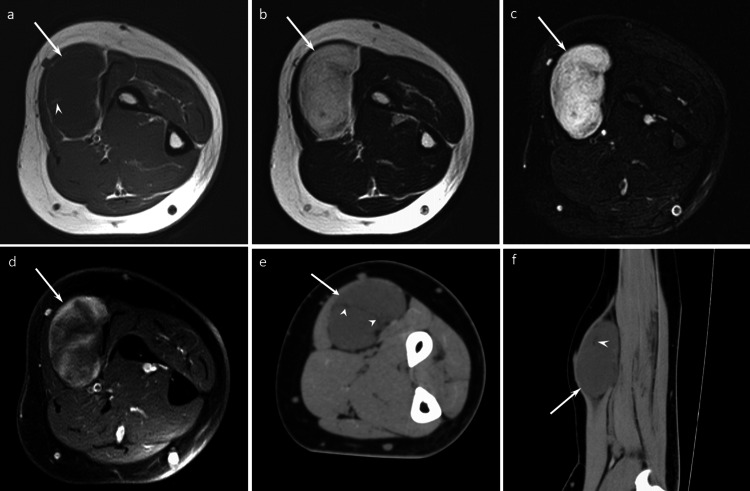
A 55-year-old man with an ASCPLT in the left forearm Axial T1WI (a), axial T2WI (b), and axial FST2WI (c) demonstrate a well-defined intermuscular non-fatty mass (arrows) with septal/linear fatty components (arrowhead) and no capsule. The non-fatty components display marked hyperintensity on T2WI. Axial FSCET1WI (d) shows nodular heterogeneous enhancement. Axial CT (e) and Sagittal CT (f) show a well-defined intermuscular non-fatty mass (arrow) containing a small fatty area (arrowhead). The imaging findings are consistent with myxoid liposarcoma pattern.
